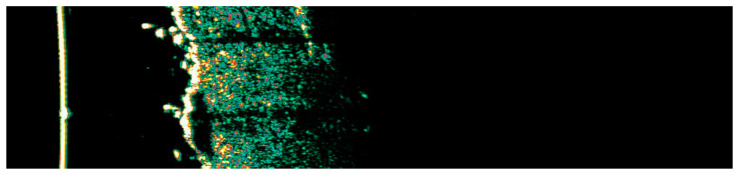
Ultrasonographic image of BCC on the back.
